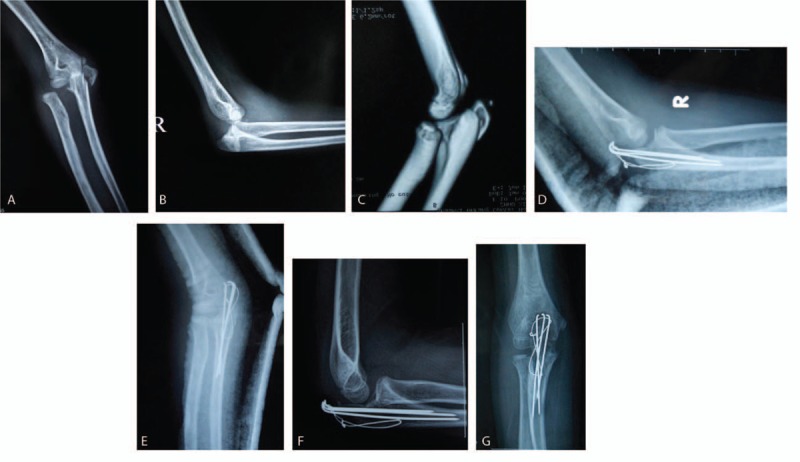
10-year-old girl, preoperative anteroposterior film showed right olecranon and coronoid fractures, and the proximal radioulnar separation and displacement (A), lateral radiographs showed right elbow posterior dislocation (B); after close reduction, CT showed the correction of right elbow dislocation, the good reduction of coronoid fracture, and a large displacement of olecranon fracture (C). Postoperative X-ray showed the good reduction of fracture-dislocation and reliable fixation (D, E); 1 year after surgery, x-ray showed that right lateral elbow dislocation was well reset, and fracture healed (F, G). CT = computed tomography.
